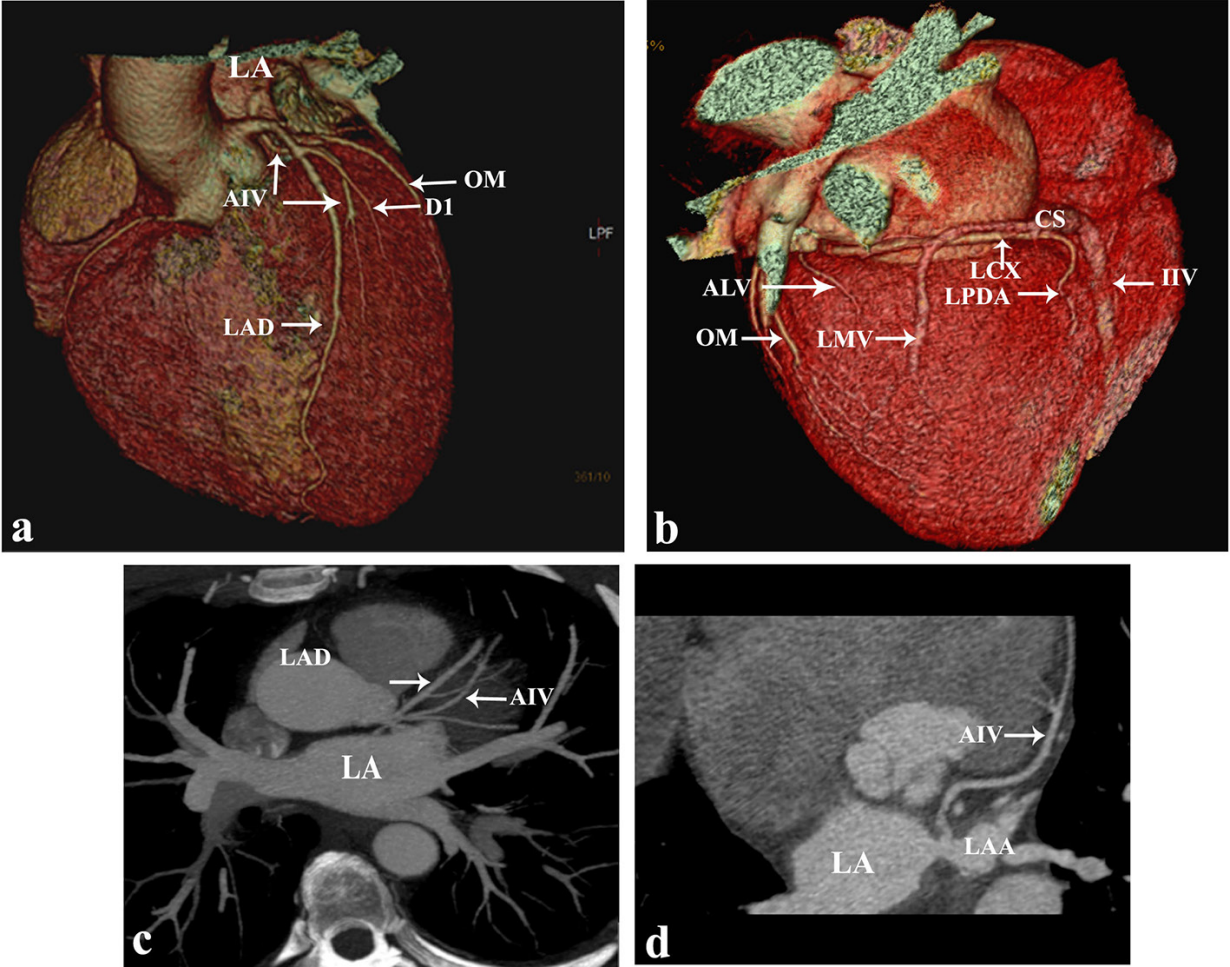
Multidetector coronary CT: three-dimensional volume rendering images (a, b). Oblique maximum intensity projection (MIP) image (c). Curved reformatted image (d). AIV passes parallel to LAD in the anterior interventricular sulcus deep to diagonal and left main arteries into the left atrium. Between AIV & IIV, there is ALV & LMV. AIV:anterior interventricular vein; CS: coronarysinus; D1: first diagonal artery; IIV: inferiorinterventricular vein; LAA: left atrial appendage; LA: left atrium; LAD: left anterior descending artery; Lcx: left circumflex artery; LPDA: left posteriordescending artery; OM: obtuse marginal artery; MIP: maximumintensity projection.
